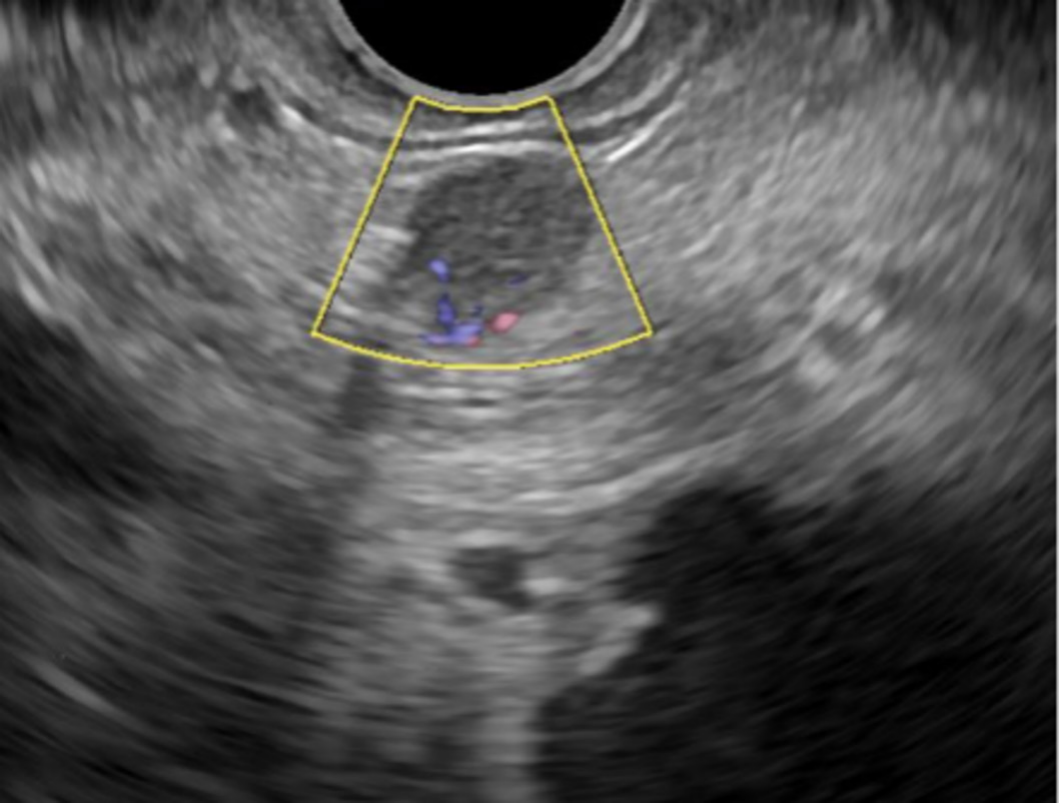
EUS color Doppler view demonstrates a well‐circumscribed hypoechoic nodule in the pancreas showing intralesional bidirectional vascular signals of a small hypervascular, nonfunctioning PanNET.
